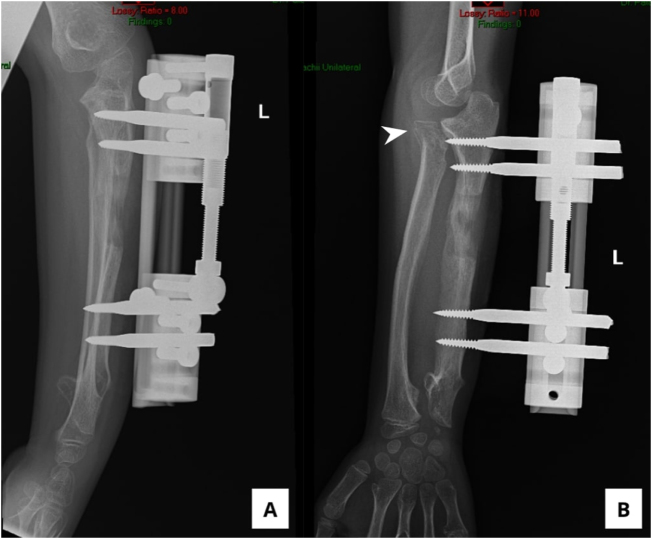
Two months post-operative plain radiograph of left forearm (A) AP view shows callus bridging; (B) lateral view shows radial head reduction (white head arrow).
